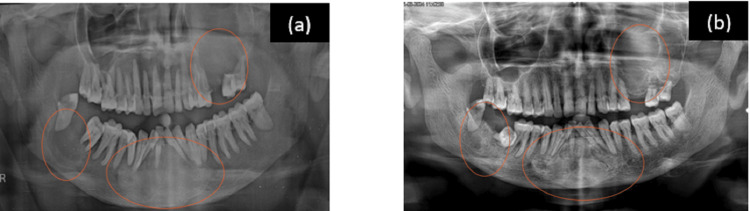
Orthopantomogram (OPG) (a) OPG taken at 6 months post-op showing increased radiopacity in the mandibular symphyseal lesion and the left maxillary region, and an increase in the size of the right posterior mandibular region, (b) all three lesions revealing an increase in radiopacity at 12 months post-op.
